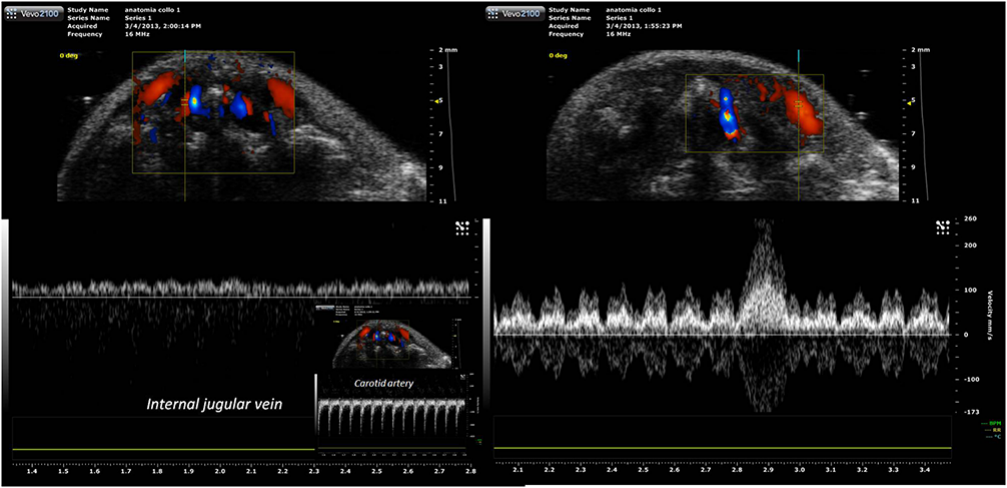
Eco-color doppler with pulsed doppler analysis of veins. (A)The internal jugular vein waveform is characterized by a monophasic pattern and low pulsatility index. (B)The external jugular vein is characterized by three-phasic pattern with high pulsatility. (C) In the small inset on the left the spectral analysis of the carotid artery blood flow is shown.
